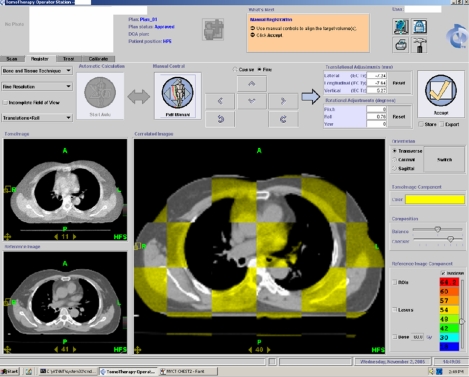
On-line treatment verification by matching of the planning CT image (bottom left) with the tomotherapy treatment set up image (top left) immediately before treatment delivery without moving the patient (courtesy of Hong Kong Sanatorium & Hospital)
